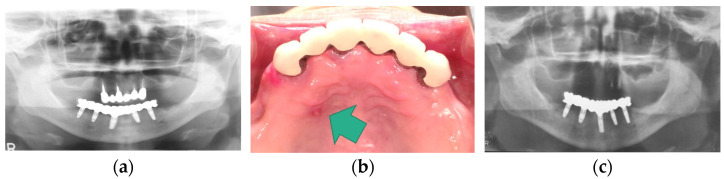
Anterior hyperfunction by an implant-supported fixed prosthesis: (a) a panoramic X-ray image at the delivery of prosthesis; (b) fistula (green arrow) attributed to root fracture; (c) a panoramic X-ray image after the loss of maxillary anterior teeth due to anterior hyperfunction by mandibular implant-supported prosthesis. Source: the authors.
